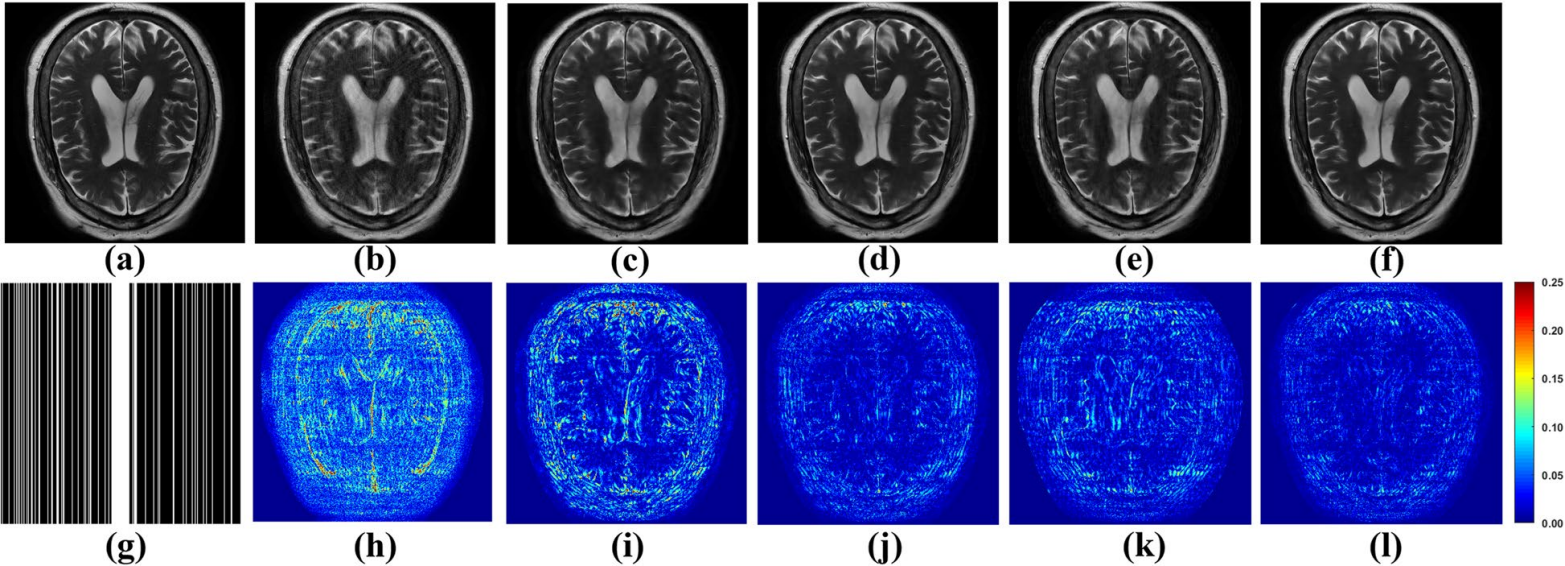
The proposed GCESS network compared with state-of-the-art MRI reconstruction methods. a Is the fully sampled image. The experiments correspond to a 1D Cartesian sampling with AF = 4 as shown in (g). b-f Are the images of reconstructed results by SPIRiT, IUNET, DCCNN, MoDL, and GCESS, respectively. h-l Are the corresponding error maps. The PSNR of (b-f) are 28.67, 29.44, 33.33, 32.15 and 34.61 dB, respectively
